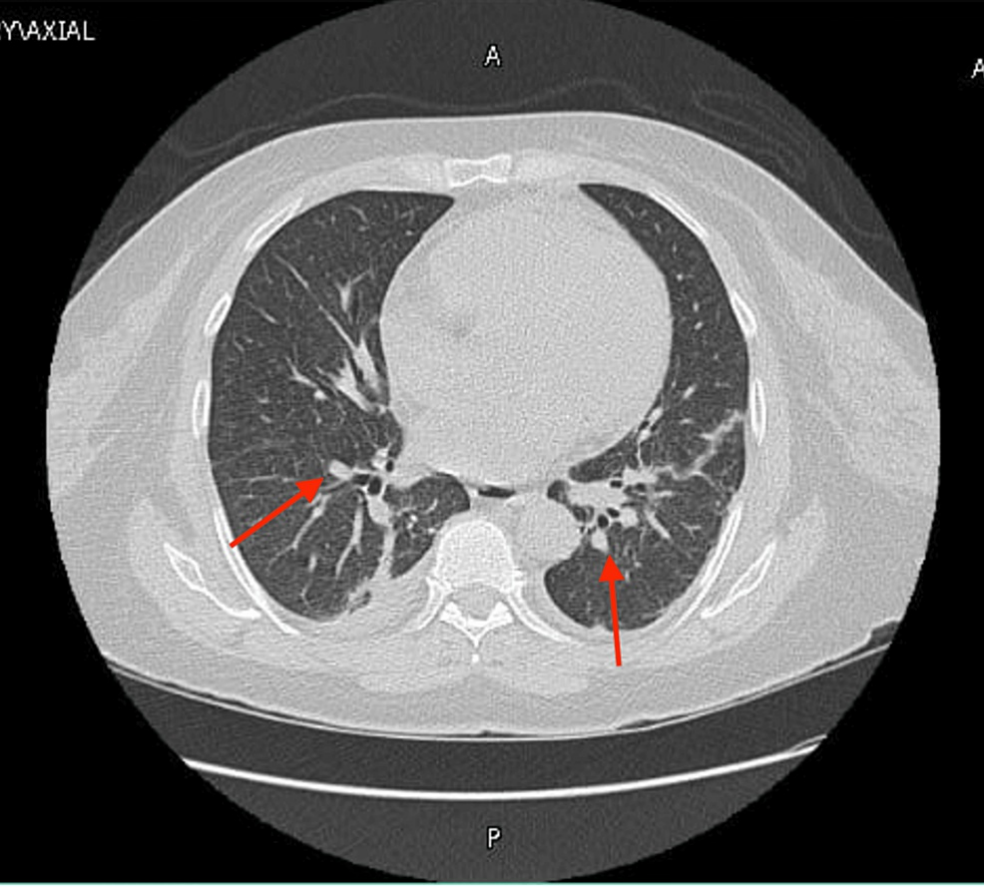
CT chest revealing multiple subcentimeter nodules Red arrows point to some of the nodules
